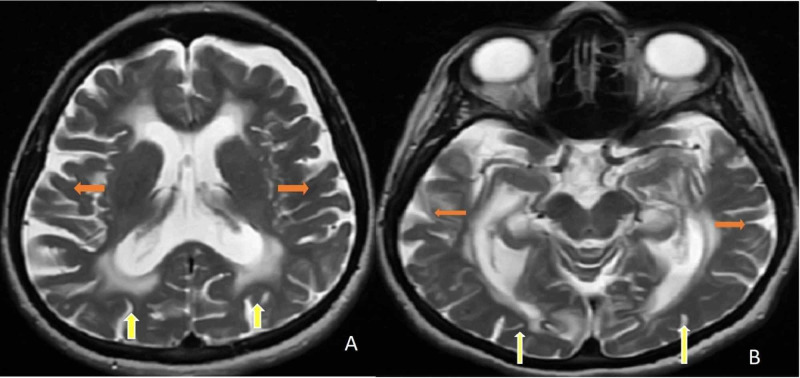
A-B. Axial T2 brain MRIs demonstrate bilateral periventricular, subcortical frontal, and parieto-occipital white matter involvement (yellow arrows). Axial T2 brain MRIs show atrophy of the cerebrum (orange arrows) with hyperintensities in the bilateral periventricular and subcortical white matter (yellow arrows). MRI: magnetic resonance imaging
